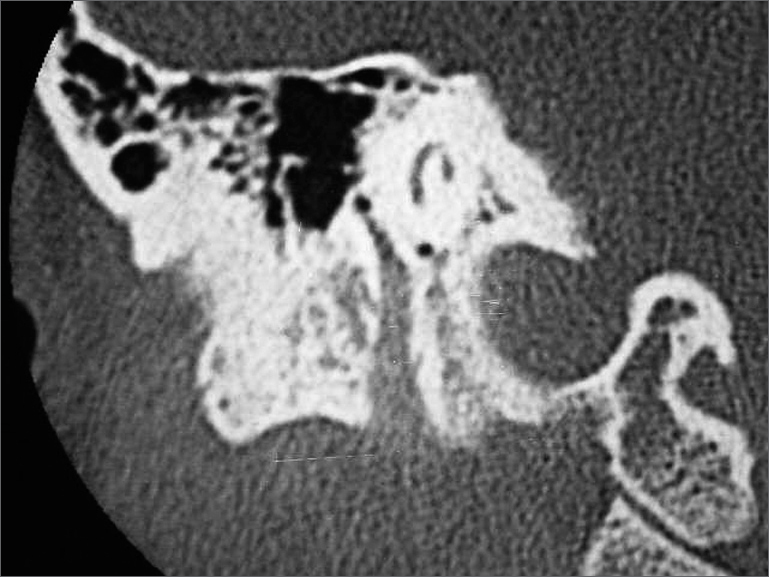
Coronal temporal bone CT scan, showing an image with soft tissue density, occupying and enlarging the third portion of the facial nerve, all the way to stylo-mastoid foramen.
